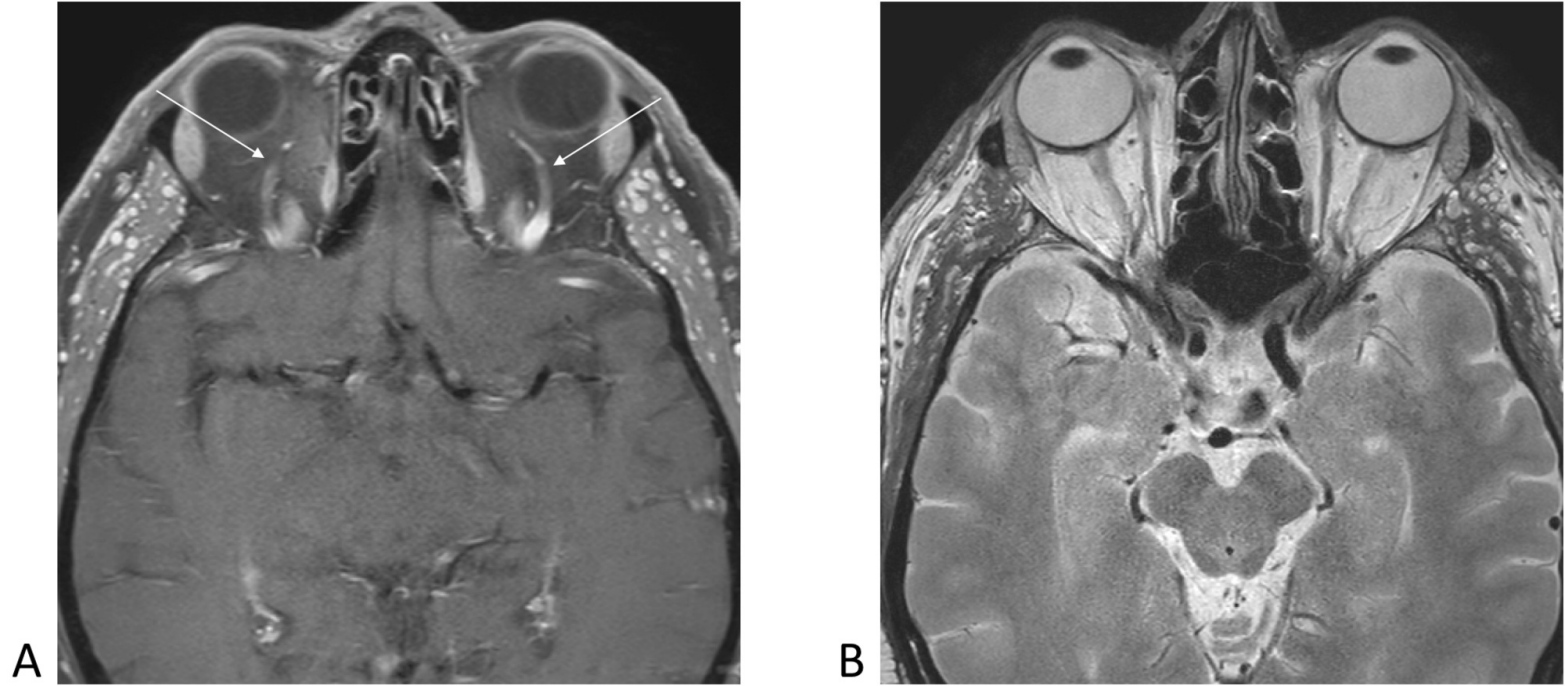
Axial magnetic resonance images demonstrating normal appearance of bilateral superior ophthalmic veins (arrows) and normal morphology of eyeballs and optic nerves bilaterally. 3A, axial T1-weighted with contrast. 3B, axial T2-weighted without contrast.
